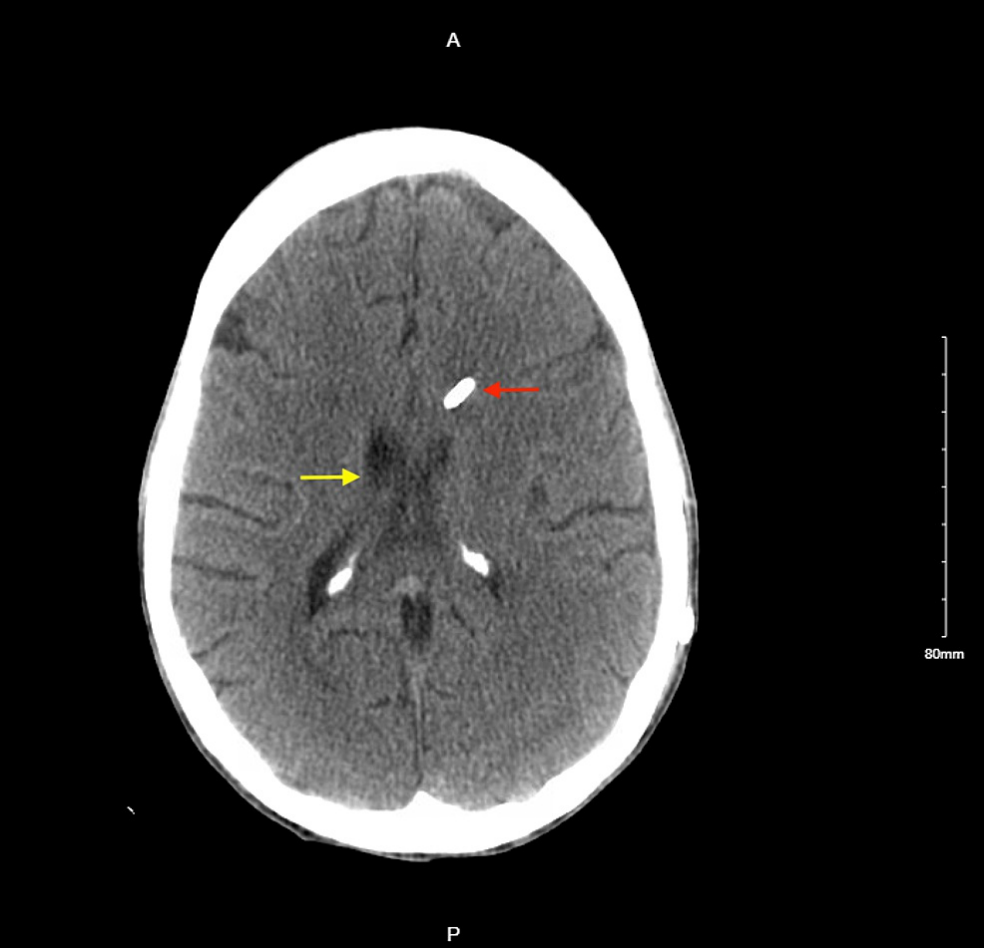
CT head obtained after the creation of ventriculoperitoneal shunt: interval placement of the left ventriculoperitoneal shunt and interval removal of the right ventriculoperitoneal shunt (red arrow), no ventriculomegaly seen (yellow arrow).
